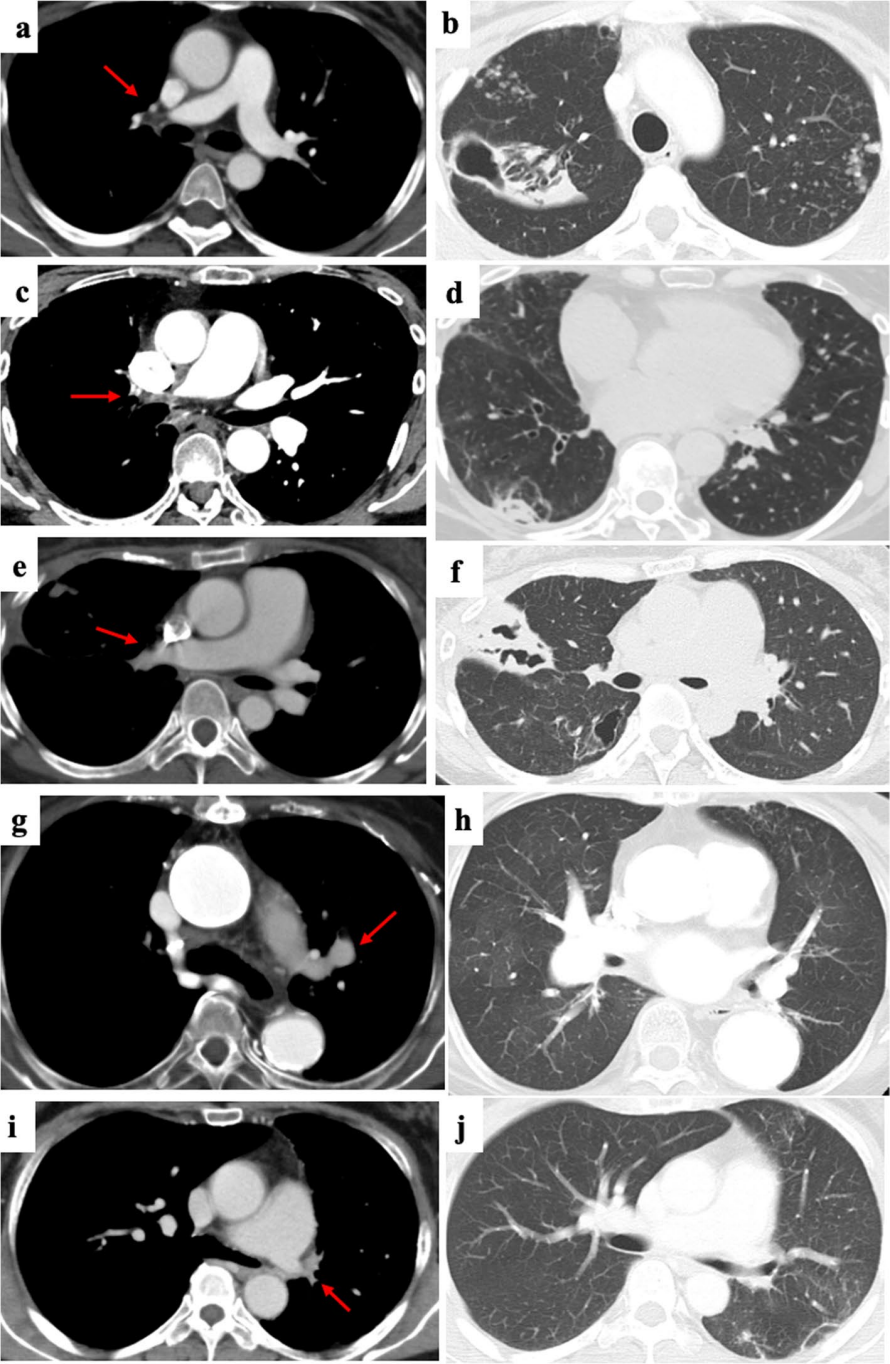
Pulmonary artery involvement (PAI) and nontuberculous mycobacteria (NTM) disease imaging on computed tomography. Patient 1: occlusion of the right upper and middle pulmonary arterial branches (a) and a large opacity with a cavity in the right lower lobe and ill-defined nodules in bilateral lungs (b). Patient 2: occlusion of the right main pulmonary artery (c) and a large opacity in the right lower lobe (d). Patient 3: occlusion of the right upper and middle pulmonary artery branches (e) and a large opacity with a cavity in the right lung (f). Patient 4: dilation of the left pulmonary artery branches (g) and ill-defined nodules in the left upper lobe (h). Patient 5: occlusion of the left main pulmonary artery before developing NTM disease (i) and ill-defined nodules in the left lung (j). The left and right images of patients 2, 3, and 4 were taken simultaneously, since the previous images were not available. Patient 6 did not have PAI. Therefore, the images are not presented
